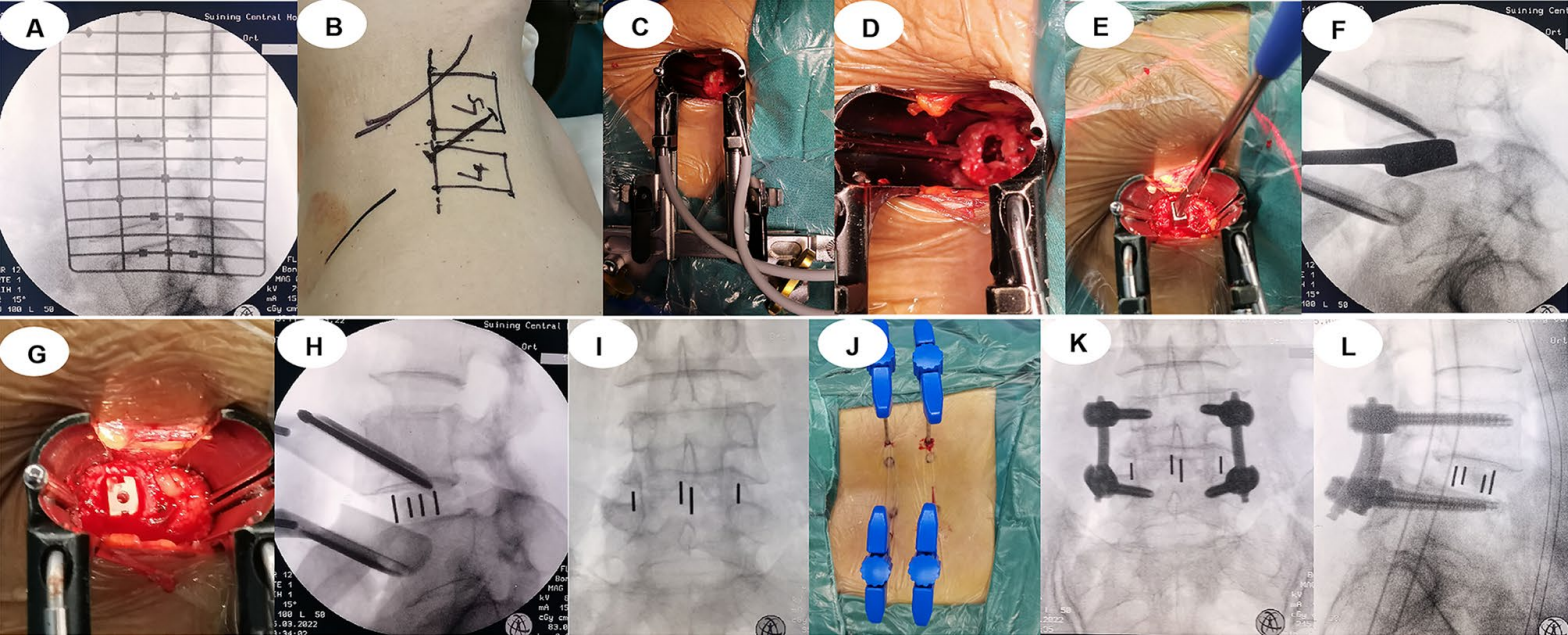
A typical OLIF case. (A, B) Marks of the L4/L5 segment and incision. (C) Exposure of the operative field and installation of the working passage. (D) Discectomy and endplate preparation. (E, F) Assessing the interbody cage. (G–I) Implantation of the cage. (J–L) Position of the cage and percutaneous pedicle screws in anteroposterior and lateral X-ray views.
